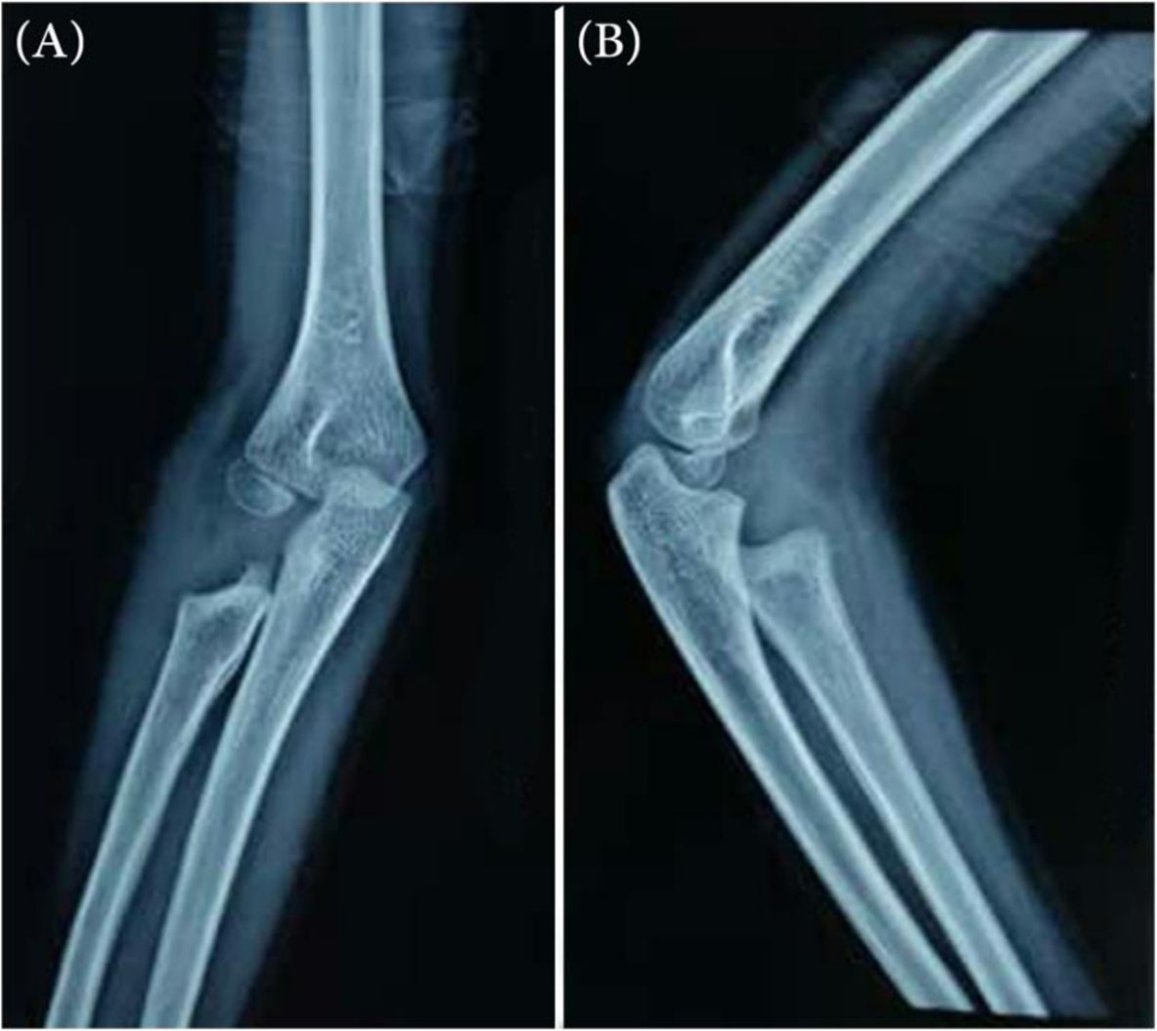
a Anteroposterior and b lateral radiograph of the elbow 1 year after the surgery showing no sign of secondary ossification or growth arrest of the forearm bone
